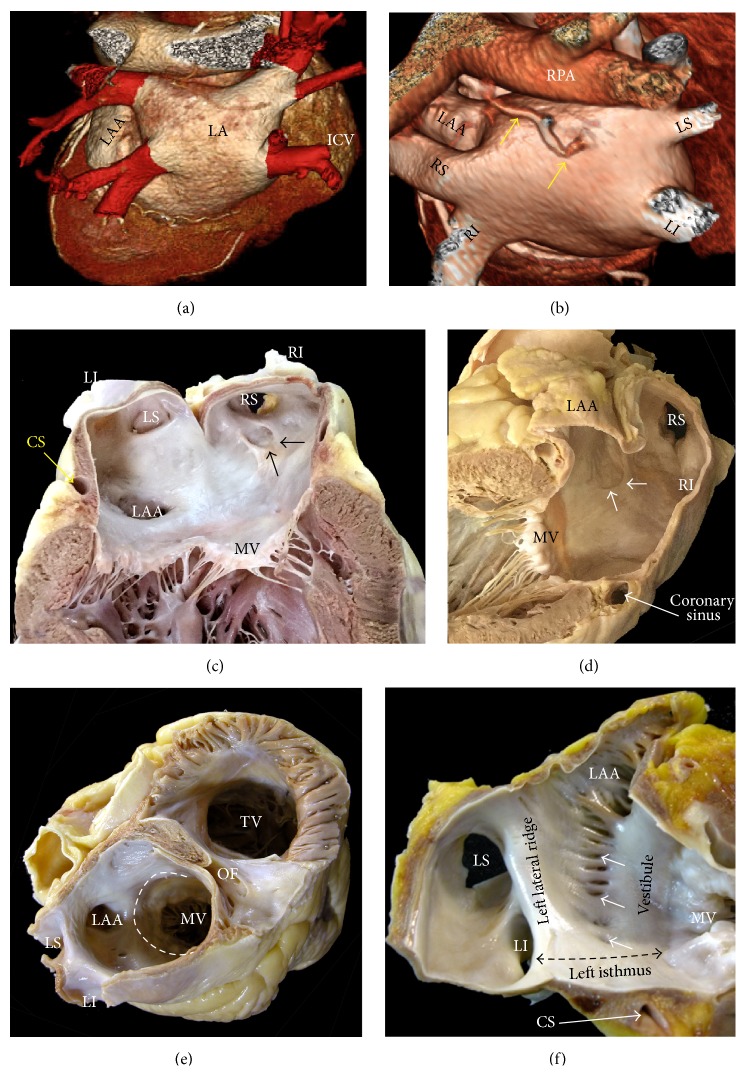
(a, b) Spatial relationship of the left atrial structures. Posterosuperior view of volume rendered CT angiographies is shown. The left atrium is located in superior and posterior aspect of the heart. (a) Normal arrangement of the pulmonary veins (in red) is shown. The left atrial appendage (LAA) is shown in yellow. (b) Again shown are normal pulmonary veins. A supernumerary pulmonary vein (yellow arrows) is shown arising from the superior wall of the left atrium. The supernumerary pulmonary vein usually drains a portion of the right upper lung and inserts directly into the left atrium body or at the junction of the right superior pulmonary vein (RS) and the left atrium. (c) Longitudinal section through the left atrium and left ventricle, showing the smooth endocardial aspect of the left atrium. The black arrows indicate the crescentic edge of the oval fossa valve. (d) Longitudinal section through the pulmonary venous component showing the orifices of the right pulmonary veins. Note the close relation of the right veins orifices with the atrial septum (white arrows). (e) Short-axis section across the atrial chambers to show the atrioventricular valves, the vestibule of the left atrium (dotted line), and the nonuniform thickness of the left atrial wall. (f) Endocardial visualization of the left posterolateral wall showing a prominent left lateral ridge and extra pectinate muscle trabeculations (arrows) extending inferiorly from the appendage to the vestibule of the mitral valve. Double-headed black broken line is showing the left atrial isthmus. CS = coronary sinus, ICV = inferior cava vein, LA = left atrium, LAA = left atrial appendage, LI = left inferior pulmonary vein, LS = left superior pulmonary vein, MV = mitral valve, OF = oval fossa, RPA = right pulmonary artery, RI = right inferior pulmonary vein, RS = right superior pulmonary vein, and TV = tricuspid valve.
